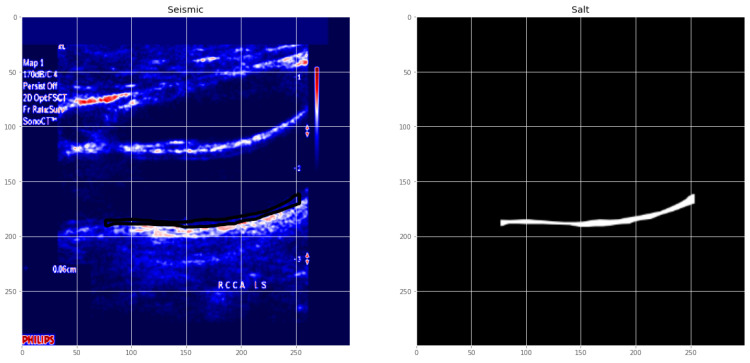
A sample of ultrasound B-mode dataset annotated for its intima–media layer mask.
